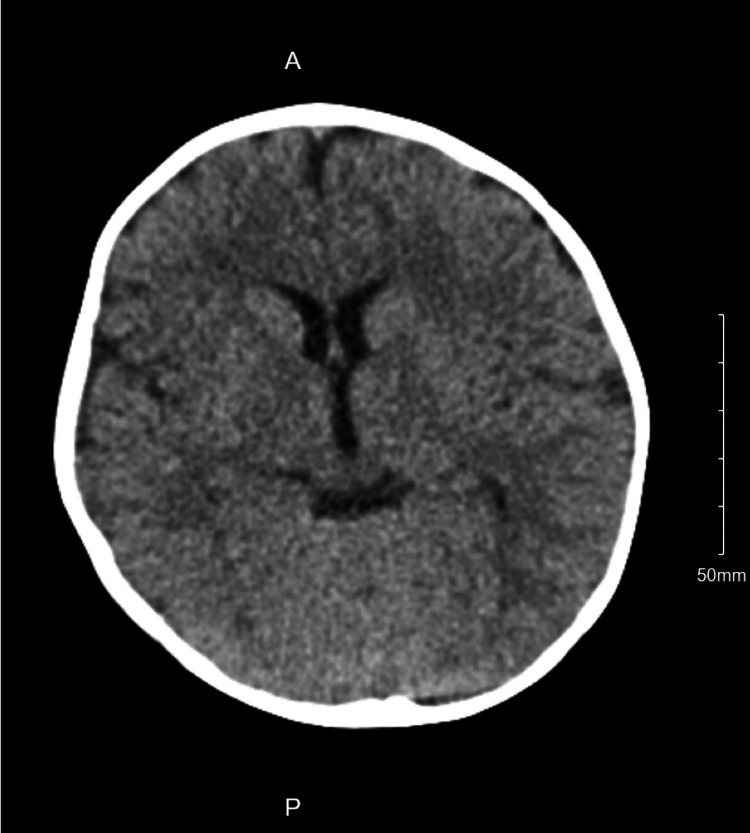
CT scan of the head showing no acute pathology CT: computed tomography
